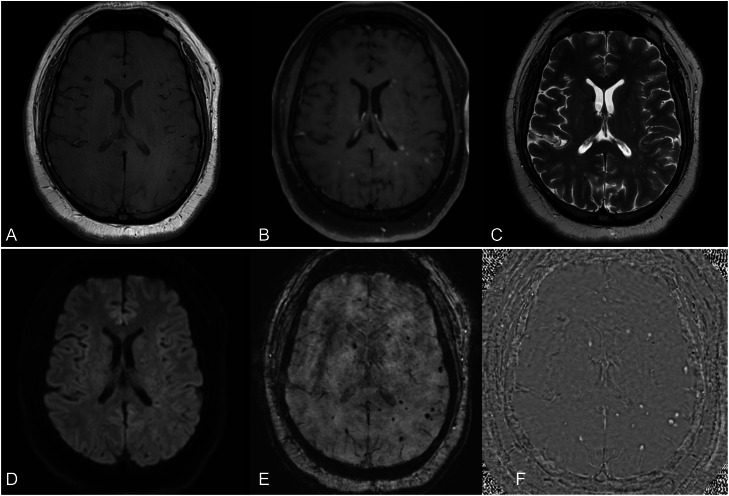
MRI head performed 3 months after presentation with axial pregadolinium contrast T1-weighted turbo spin echo image (A) & axial postgadolinium contrast T1-weighted turbo spin echo image with fat saturation (B) & axial T2-weighted turbo spine echo imaging (C), axial DWI (D), axial SWI (E) & SWI filtered phase image (F); showing a significant reduction in lesion size and resolution of perilesional edema with residual foci of hemorrhage.
